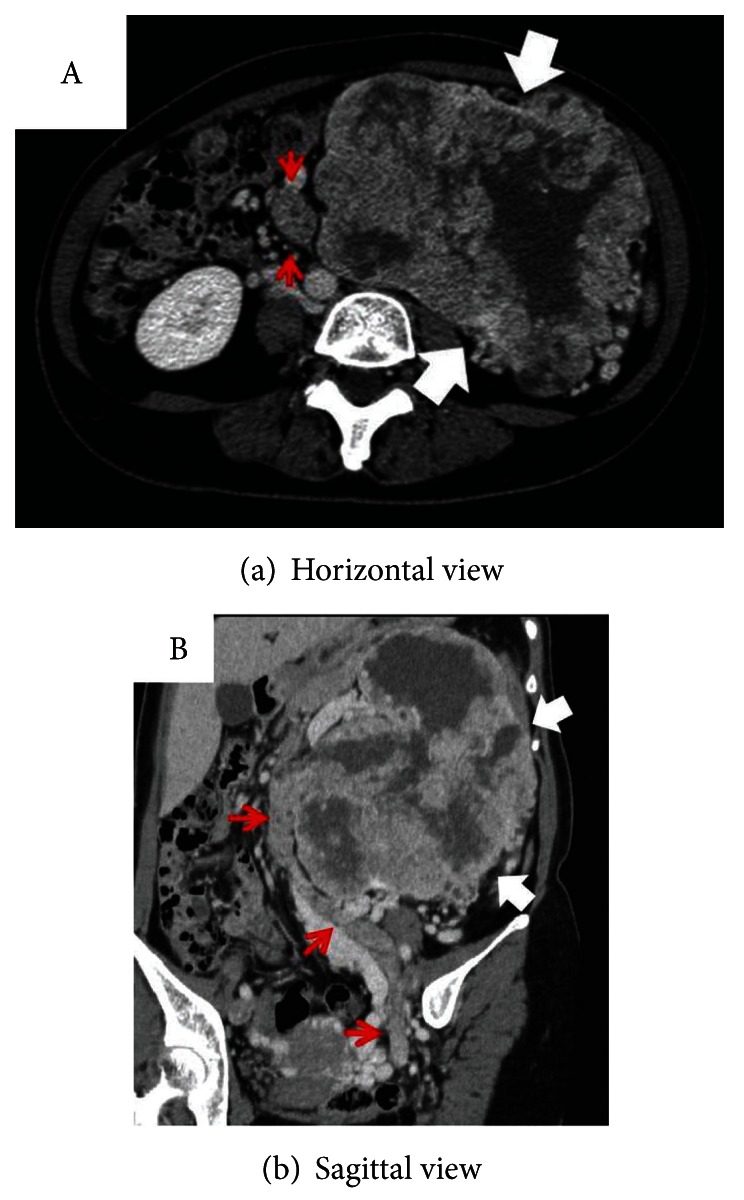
Contrast enhanced CT showed a left renal tumor with heterogenous enhancement (white arrows). Left ureter was packed with the tumor (red arrows).
